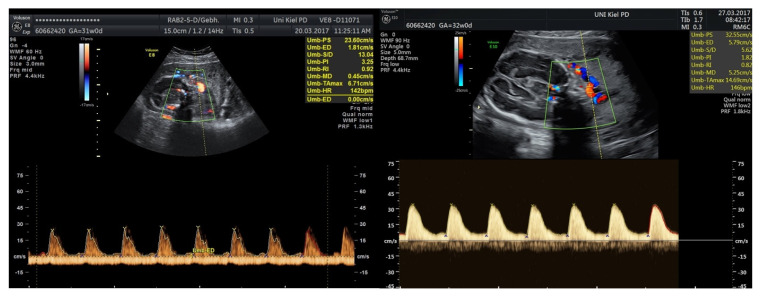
Umbilical Doppler showing absent end-diastolic flow before amnioinfusion (left), and positive end-diastolic flow (right) four days after amnioinfusion.
